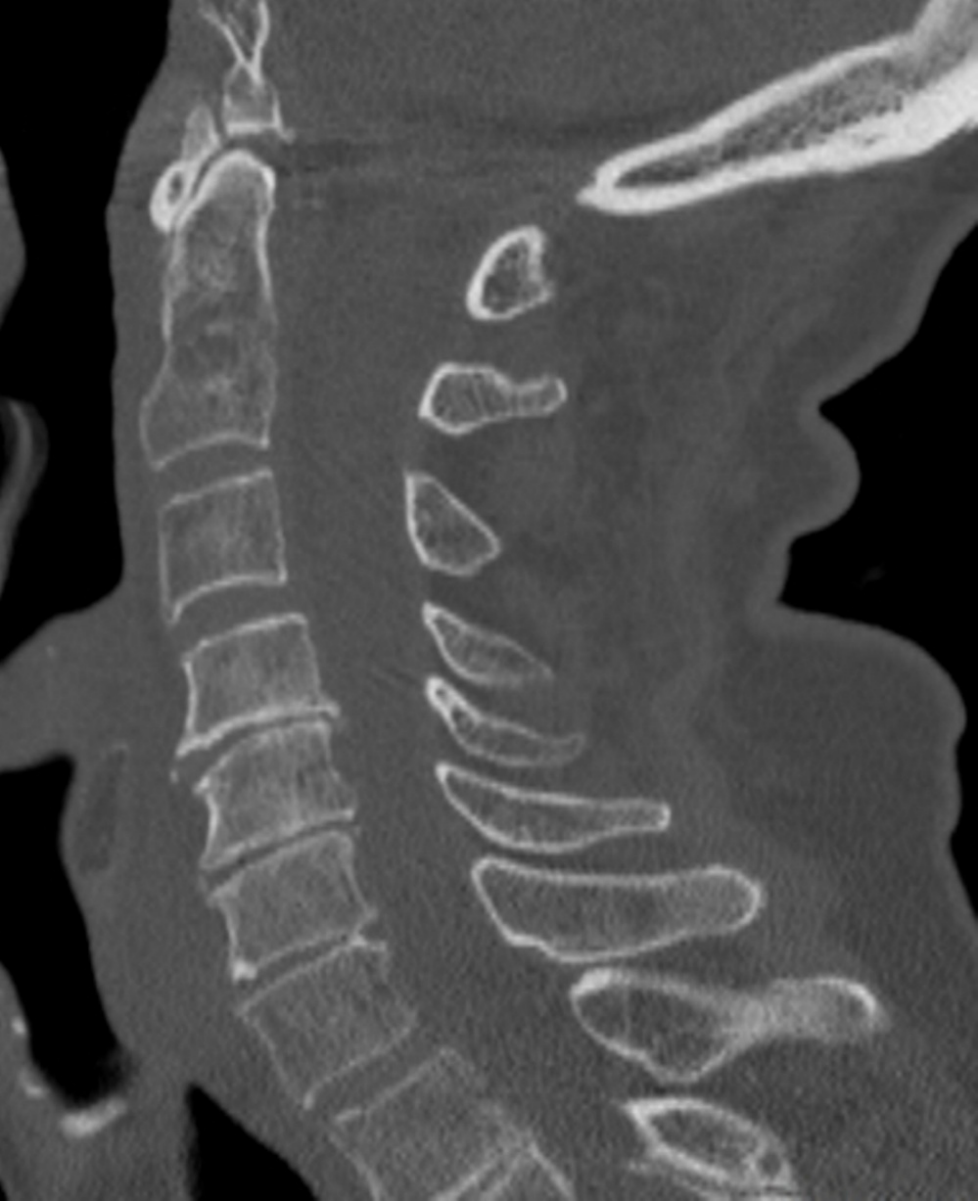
Sagittal CT of the Cervical Spine Sagittal CT image of the cervical spine showing no obvious fracture or dislocation at the time of the initial fall and evaluation by Emergency Department.
